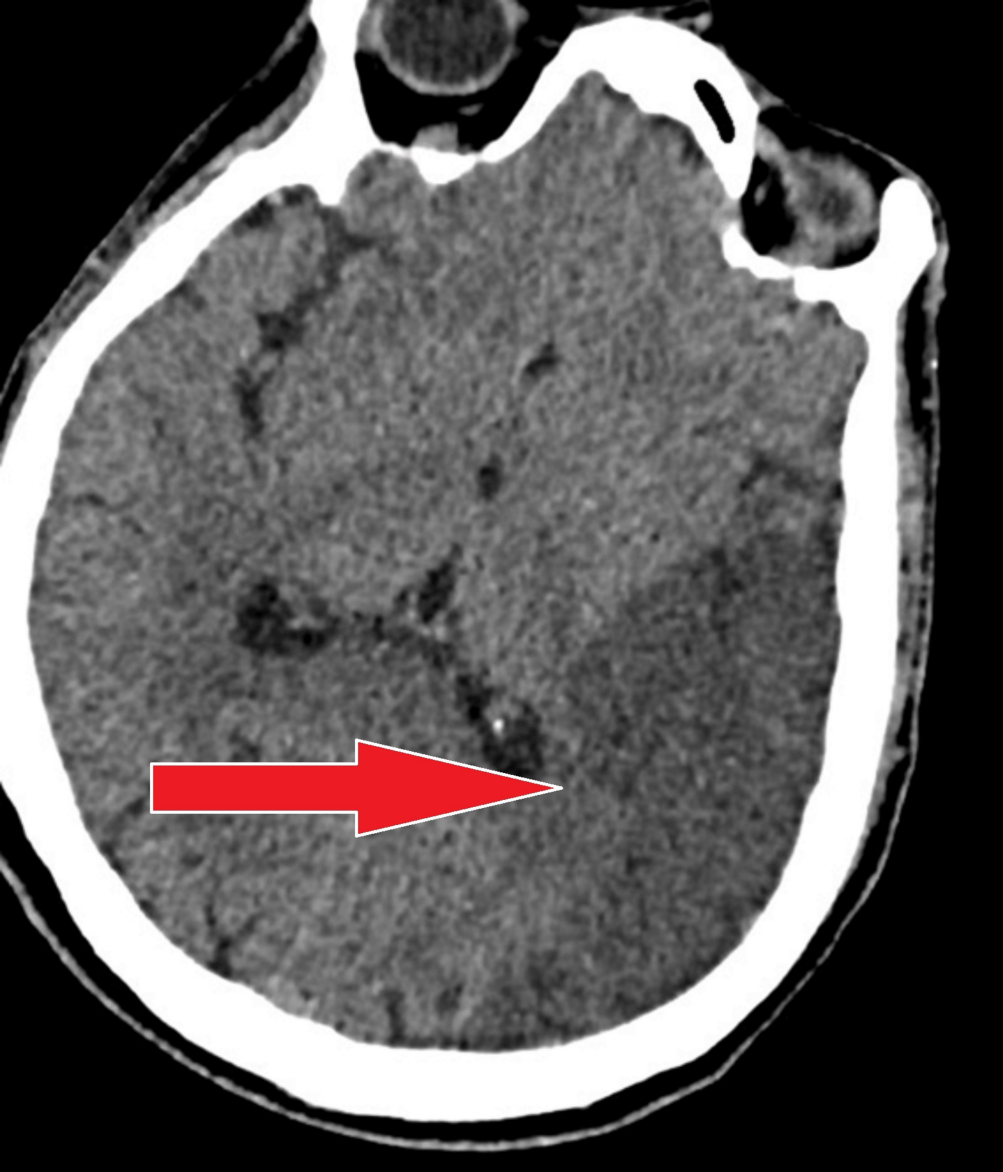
Non-contrast CT head scan showing acute and established transcortical infarcts (red arrow) in the left posterior parietal and temporal lobes. This finding confirmed an ischemic stroke involving the left middle cerebral artery (MCA) territory and guided further vascular imaging and management decisions.
